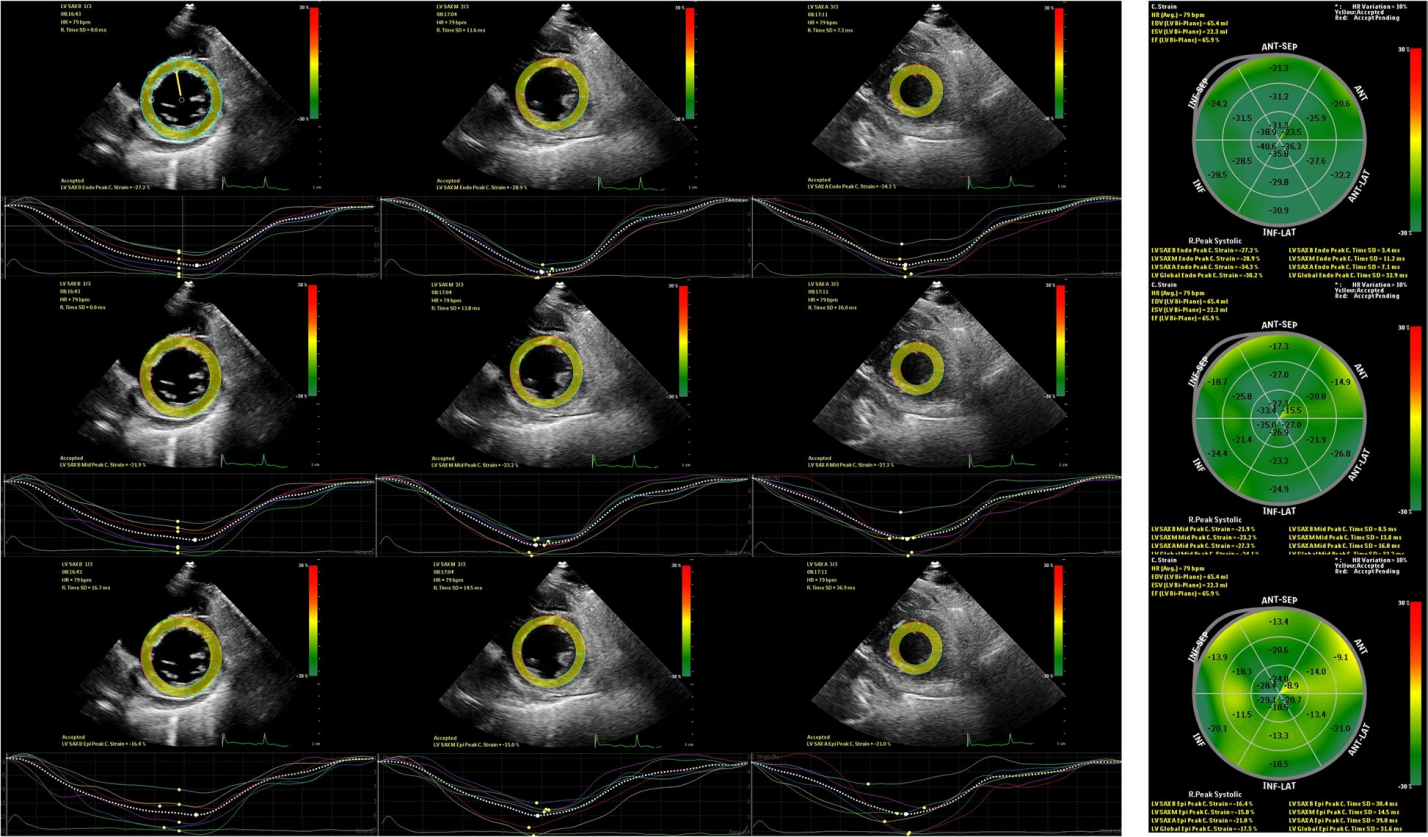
Layer-specific strain curves measurement by speckle-tracking echocardiography from a patient with SLE. LV endocardial CS (A), mid-myocardial CS (B), and epicardial CS (C) were measured from the basal, middle, and apical short-axis dynamic images
